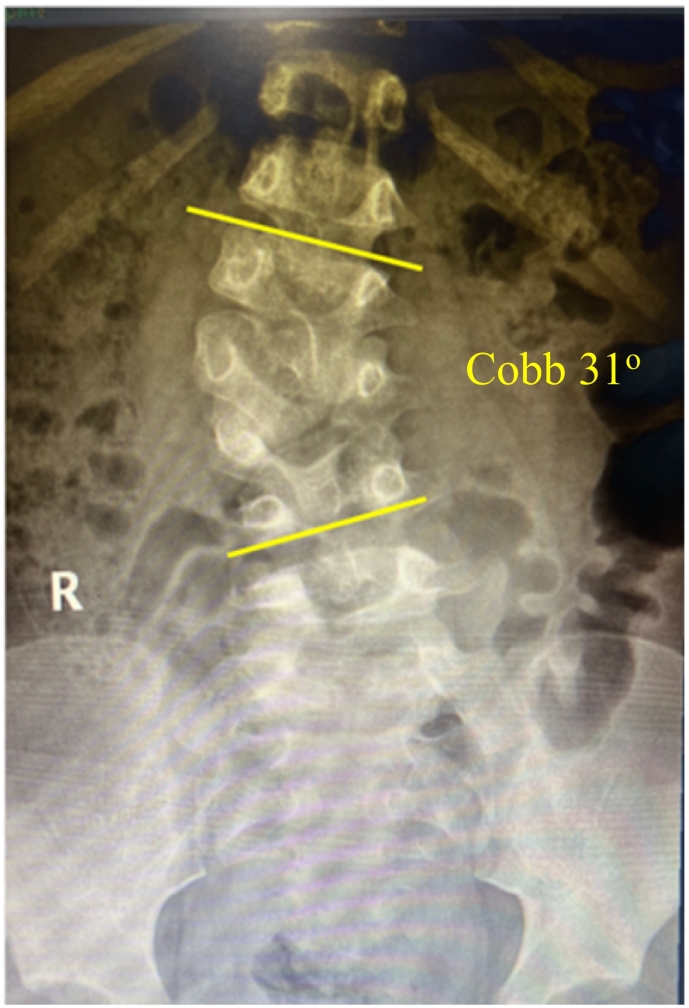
The X-ray 3 years post implantation showed lumbar scoliosis with Cobb angle of 31 degrees of lumbar.
